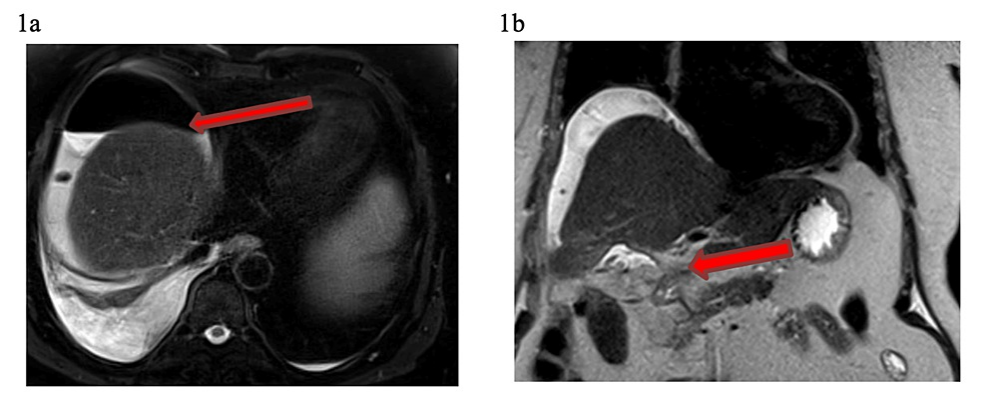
MRI without contrast. T2 fat saturation axial image demonstrates large perihepatic collection (1a). T2 coronal image demonstrated a small porta hepatis collection (1b). No intrahepatic biliary dilatation
